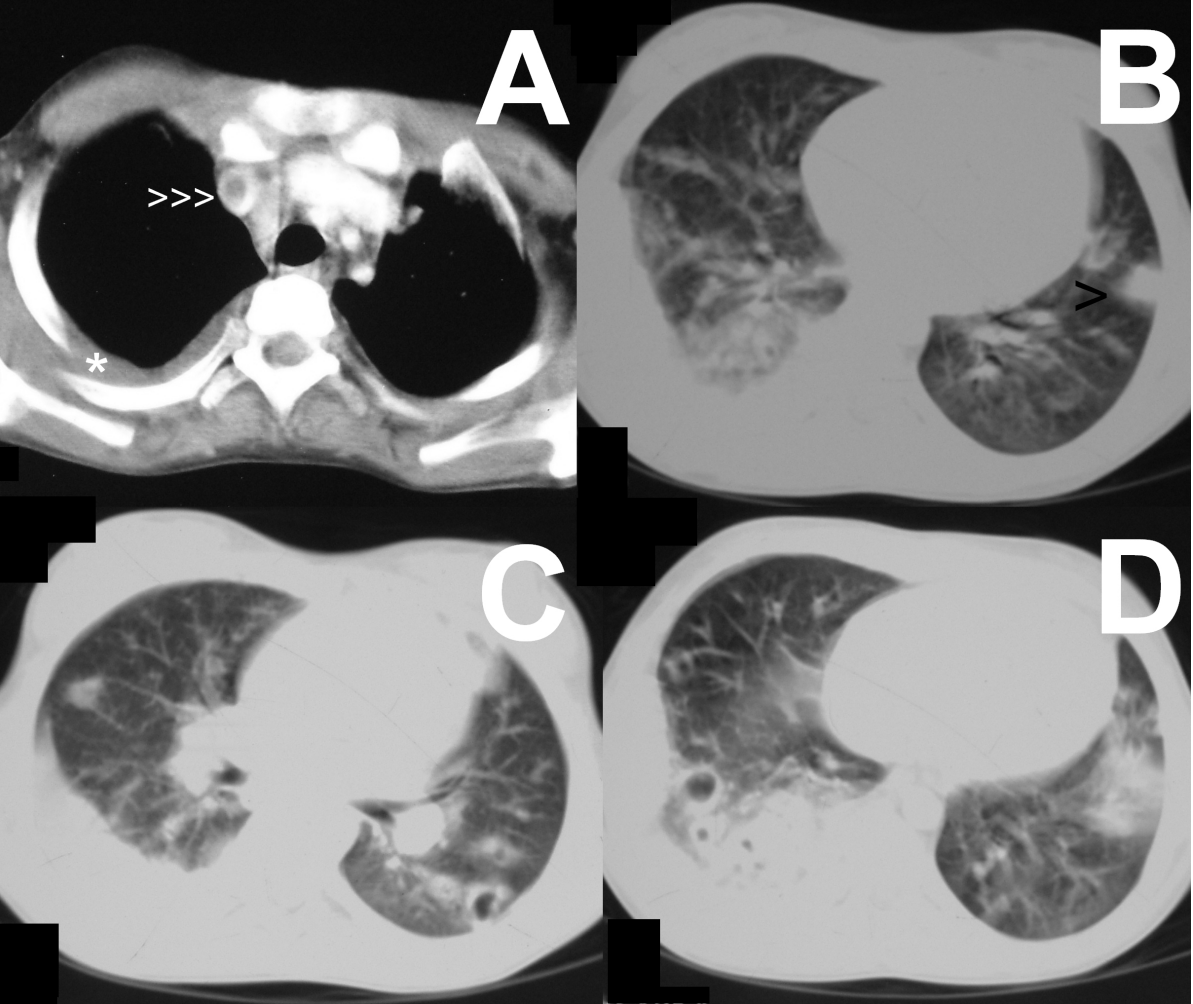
Venous thrombosis and septic pulmonary embolism. Contrast-enhanced computed tomographic images of chest showing an intraluminal filling defect caused by the thrombus in the superior vena cava (A, arrowheads), pleural-based wedge-shaped pulmonary infiltrate in left upper lobe (B, arrowhead), and cavitating infiltrates in bilateral lower lobes (C, D). Right-sided pleural effusion is also seen (A, asterisk).
